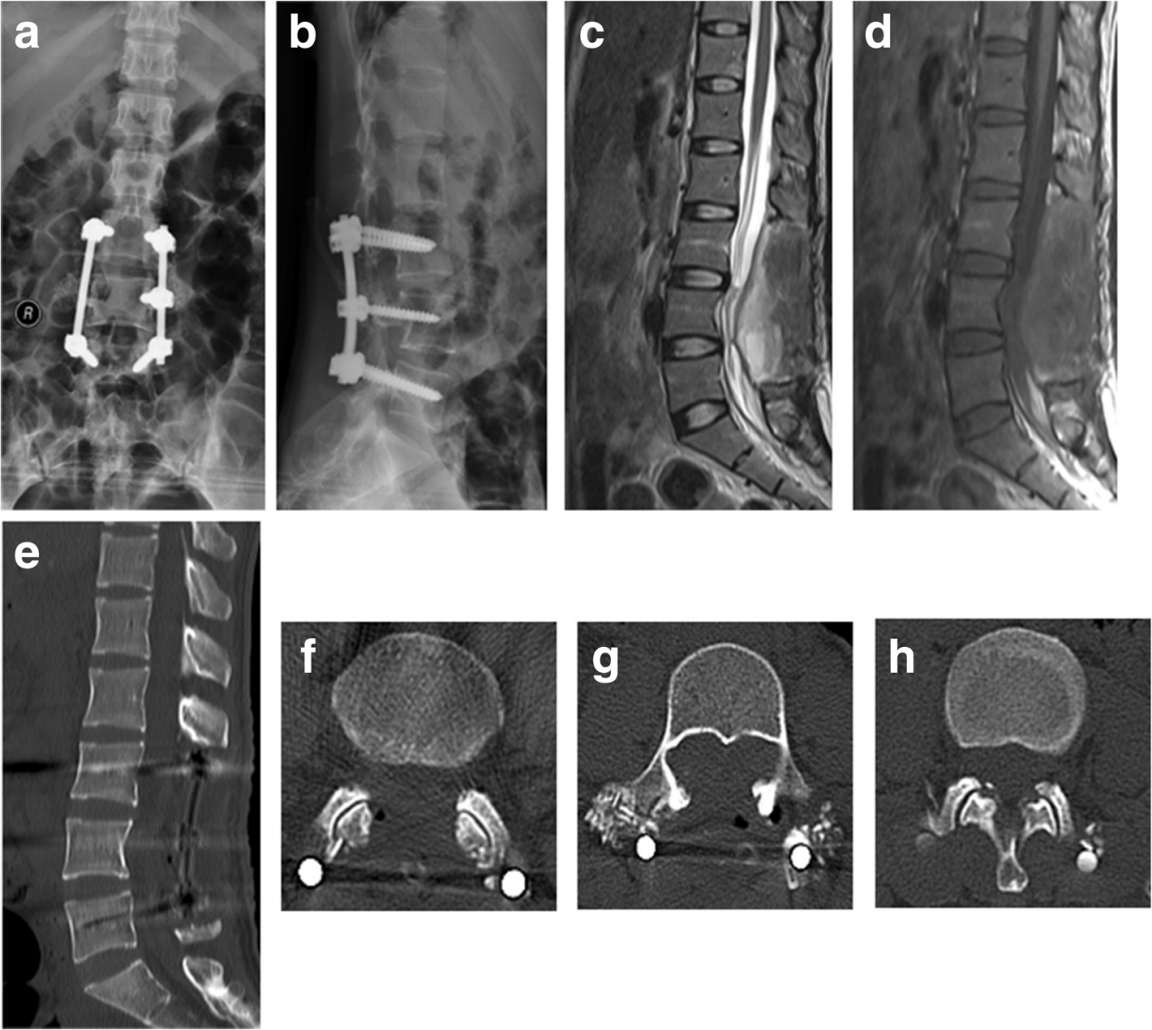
The postoperative X-rays and CT and MRI scans. (a-b) Images of postoperative X-rays. Sagittal T2WI (c) and T1WI (d) of the postoperative MRI images show the mass has been completely resected. (e-h) Images of postoperative CT confirm that the mass has been removed. e shows the sagittal plain and f-h represent the level of L3, L4 and L5, respectively
